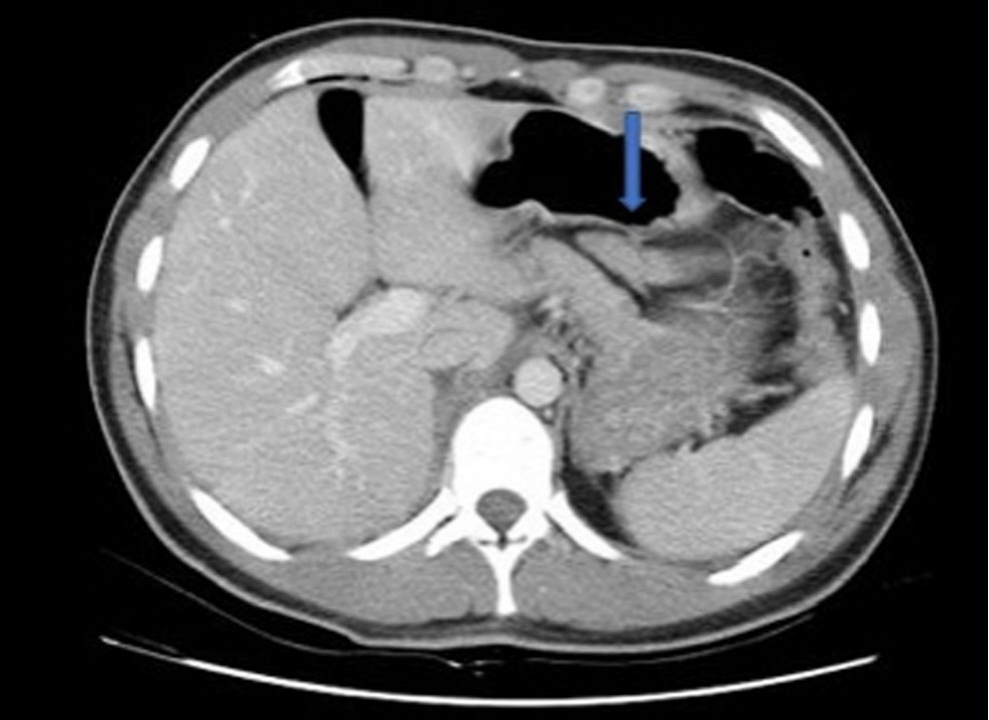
CT of a grade II pancreatic injury (blue arrow showing the injury site)
